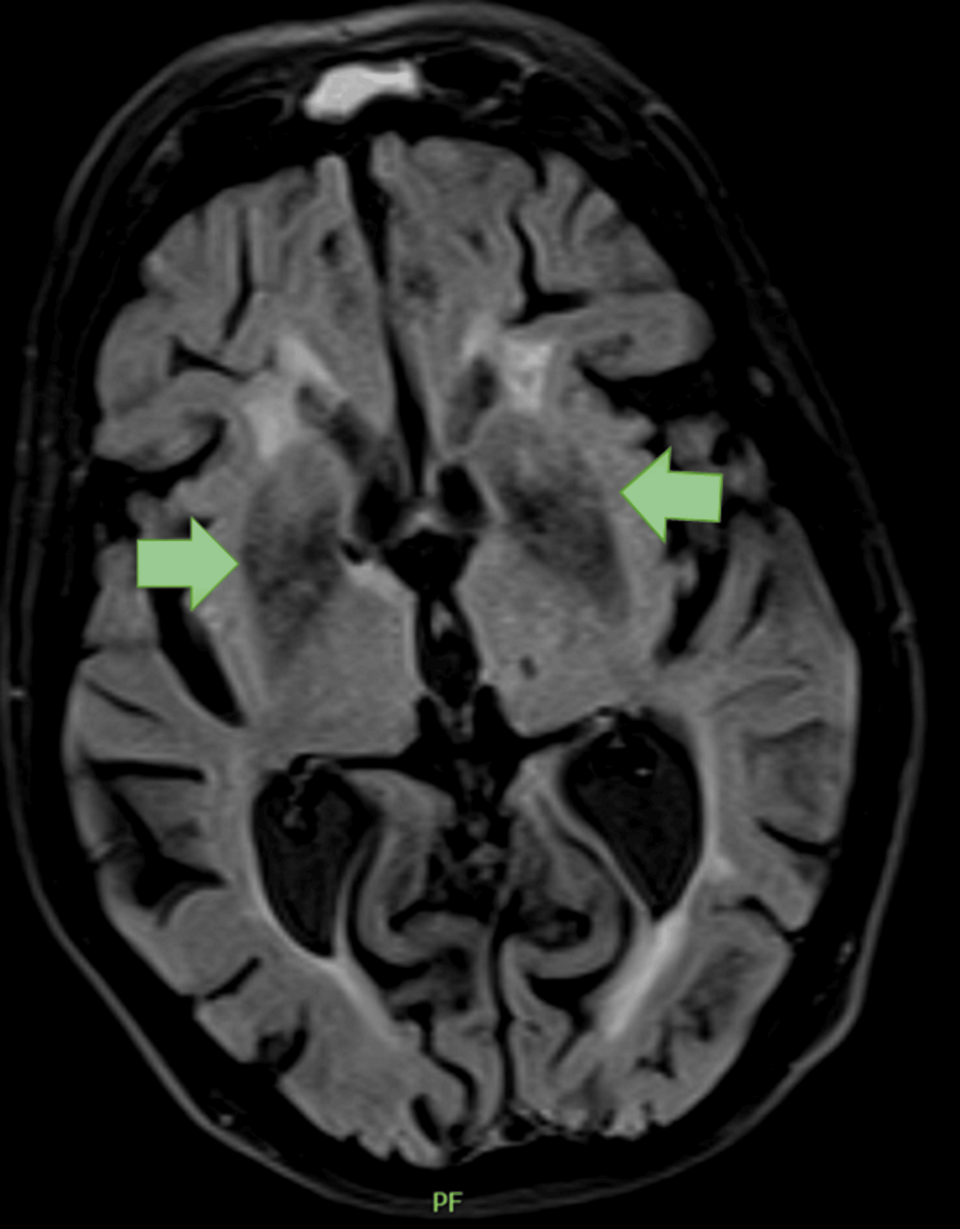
MRI of Patient 2 showing hyposignality in the corpus striatum in FLAIR T2. FLAIR: fluid-attenuated inversion recovery.
